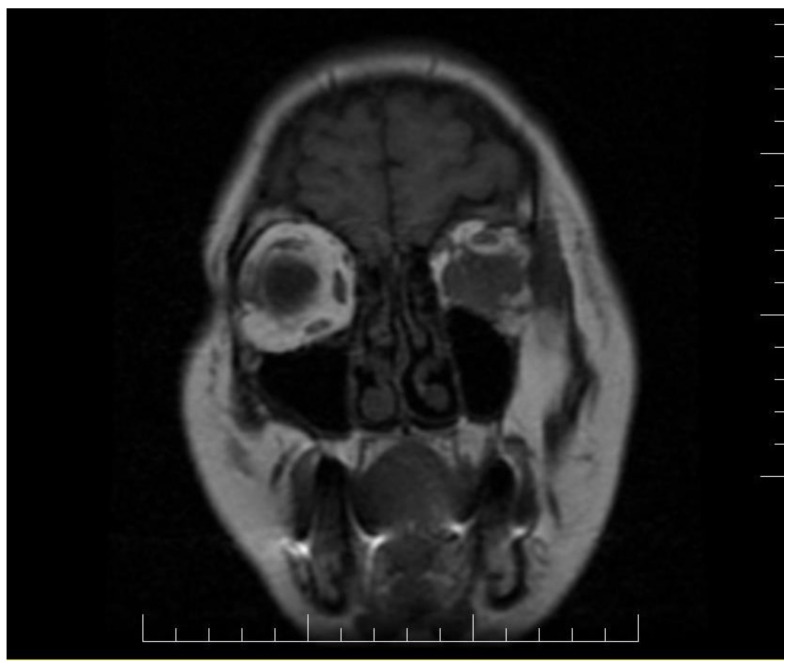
Brain MRI exhibiting a left retroocular intraorbital tumor, which infiltrated the ocular muscles in three out of quarter quadrants.
